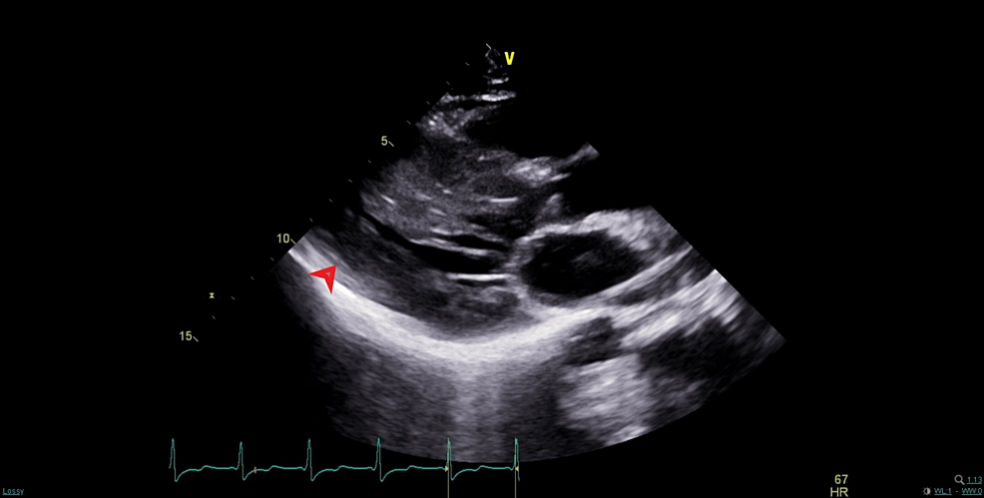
Transthoracic echocardiogram, parasternal long-axis view. The red arrow demonstrates significant left ventricular wall thickening, suggestive of hypertrophic cardiomyopathy.
